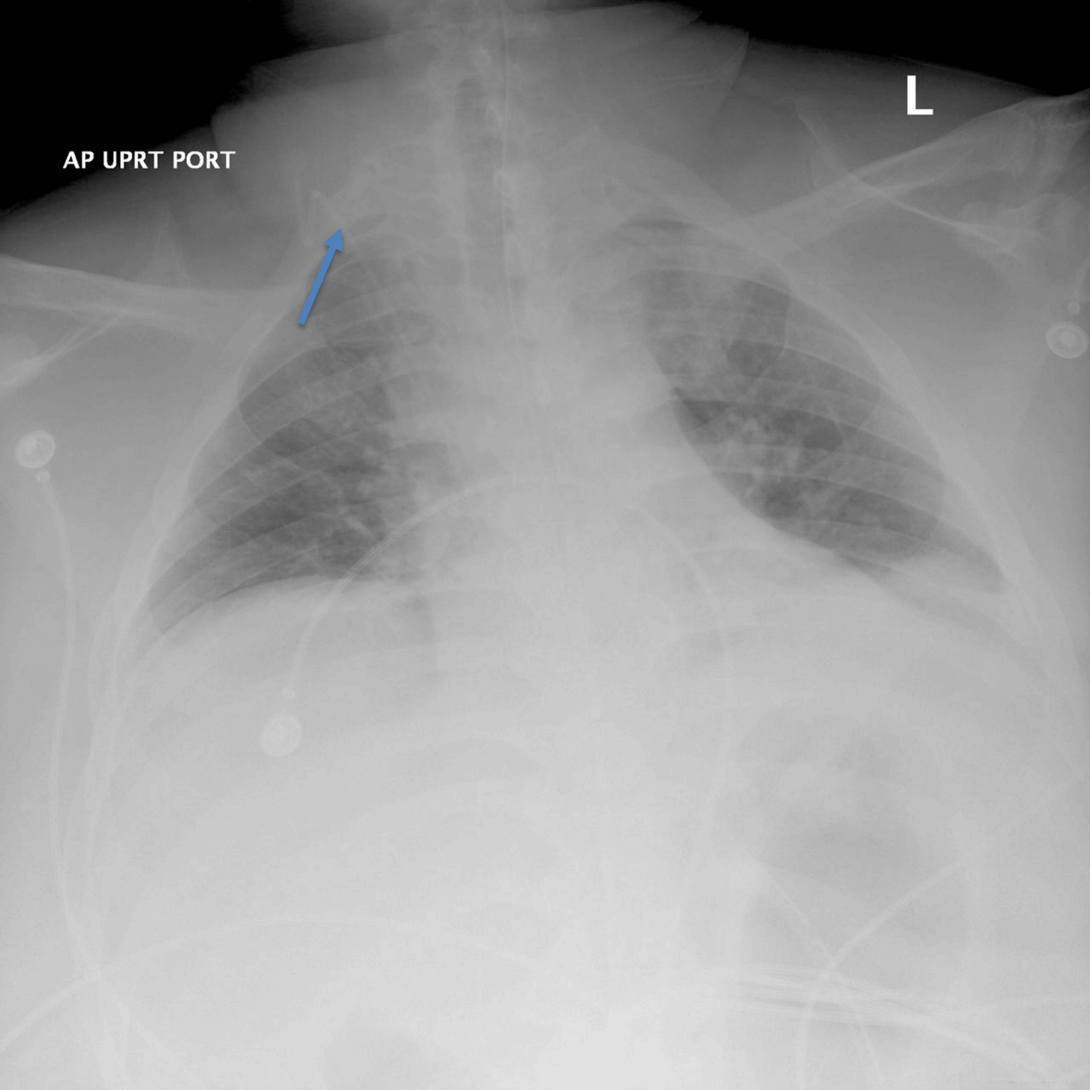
AP chest X-ray demonstrating a properly positioned right internal jugular central venous catheter The blue arrow indicates the position of the right internal jugular central venous catheter. The portable AP chest X-ray confirms the course of the CVC inserted via the right neck. The radiopaque catheter tracks inferiorly and medially, with its tip appropriately positioned within the SVC, projected just above the level of the right atrium. AP, anteroposterior; CVC, central venous catheter; SVC, superior vena cava
